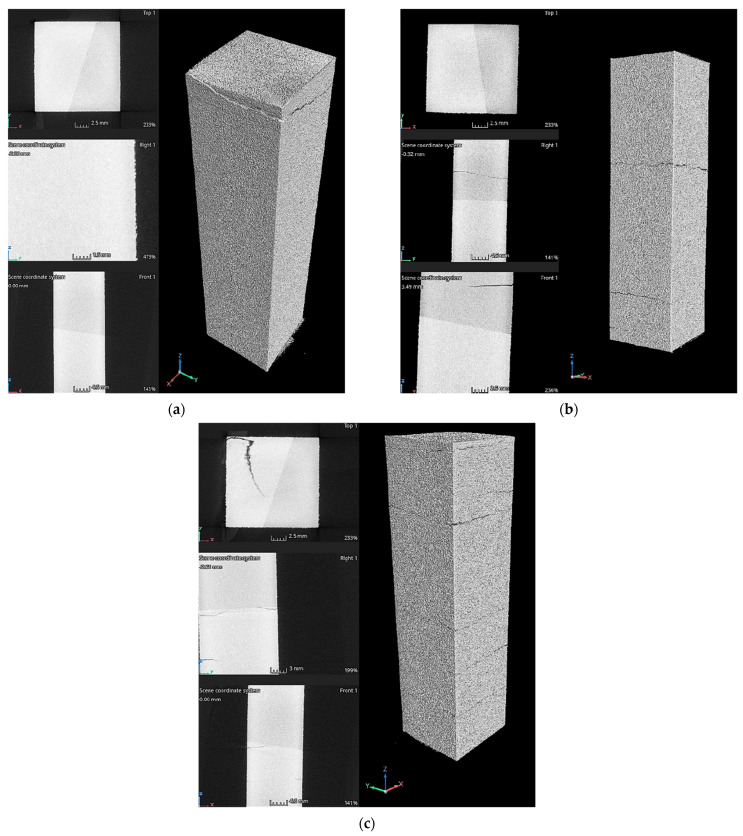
CT scans of the LPBF-printed samples after hot isostatic pressing: (a) 6XZ, (b) 7XZ, and (c) 8XZ.
